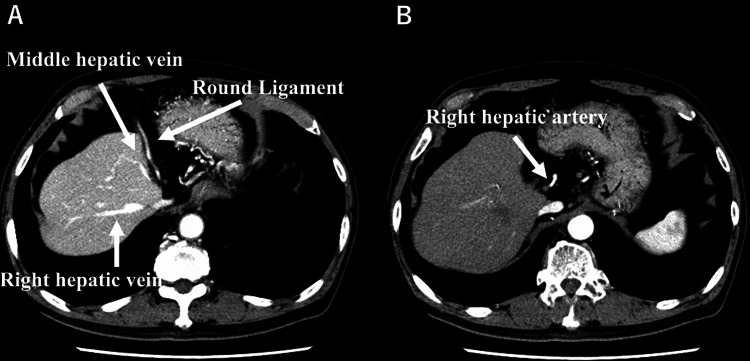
Abdominal CT scan with contrast showing (A) absence of the left hepatic lobe in the late phase and (B) absence of the left hepatic artery in the arterial phase.
